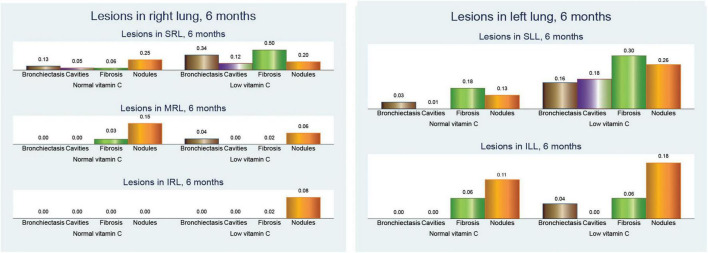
Pulmonary computed tomography (CT) lesions after 6 months of anti-tuberculosis (TB) treatment.
